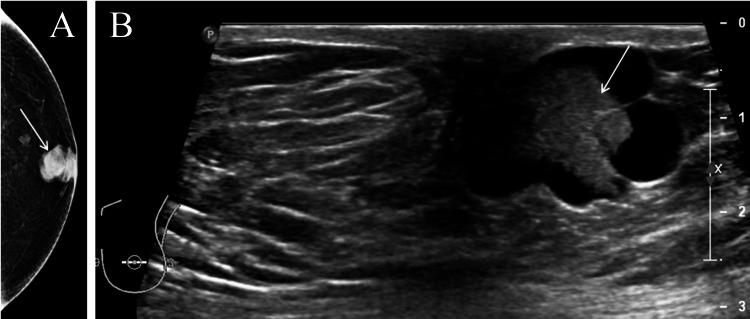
A 66-year-old male with nine months history of a breast lump (arrowed). Mammograms reveal classic a retroareolar eccentric mass shown in ultrasound as an intracystic lesion (arrowed). Pathology confirmed papillary cancer. Hormone receptor-positive and human epidermal growth factor receptor 2 (HER2) negative. (A) Mammogram - craniocaudal view. (B) Ultrasound of retroareolar region.
